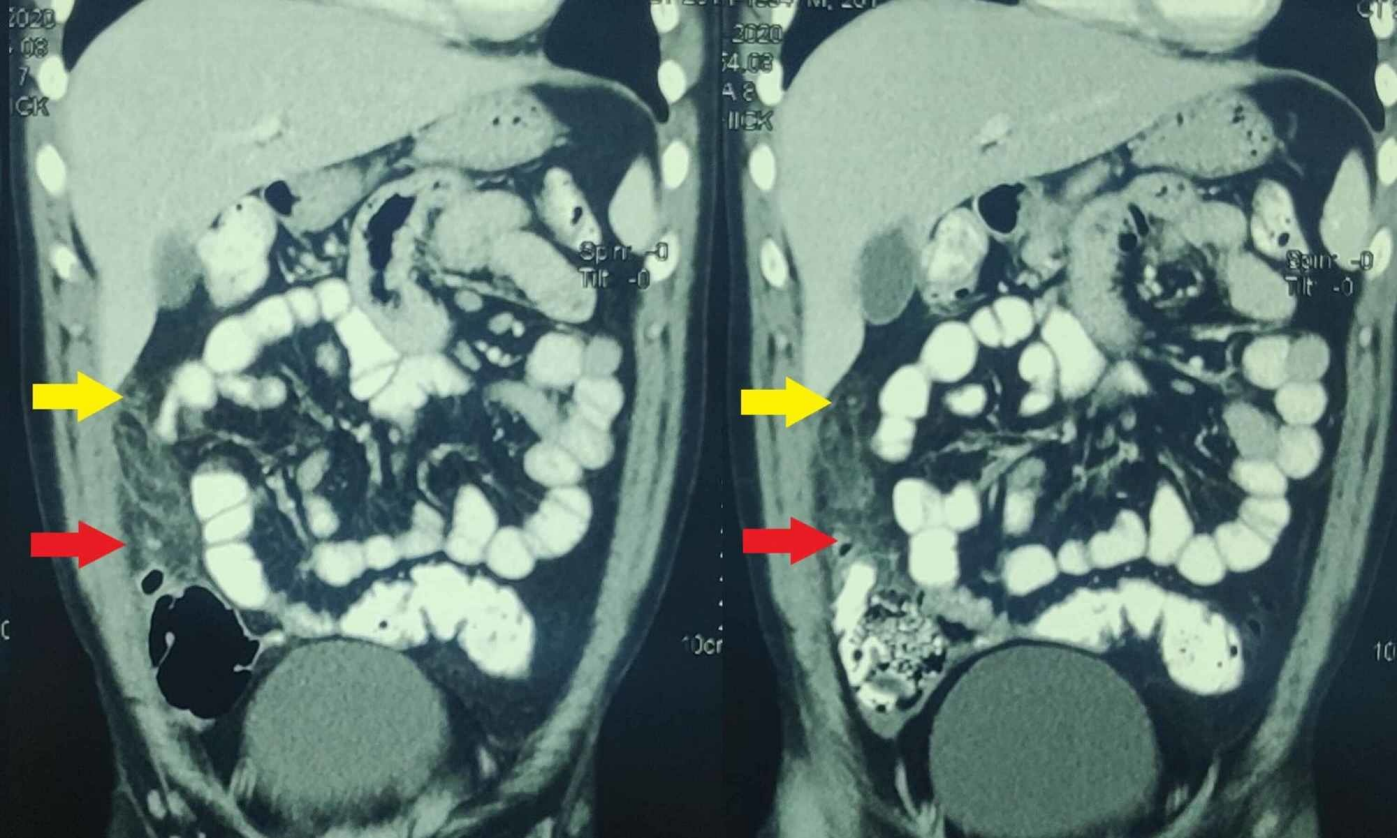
Coronal sections of contrast-enhanced CT scan of the abdomen. There is an elongated oval-shaped mixed fat and soft tissue density (red arrows) measuring 4.4 × 2.1 × 6.0 cm in the right side of abdomen, signifying omental infarction. The lesion is extending from right iliac fossa to the subhepatic region (yellow arrows).
